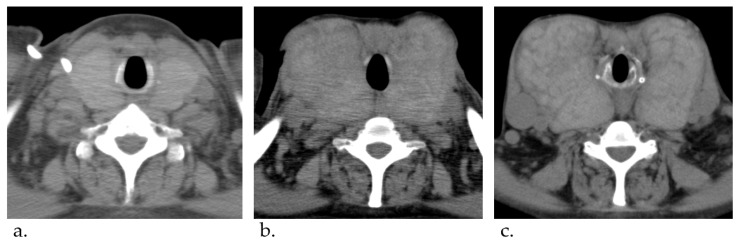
Computed tomography imaging course of thyroid gland enlargement. (a) At 45 years of age. (b) At 52 years of age. (c) At 54 years of age. The thyroid gland gradually was enlarged.
